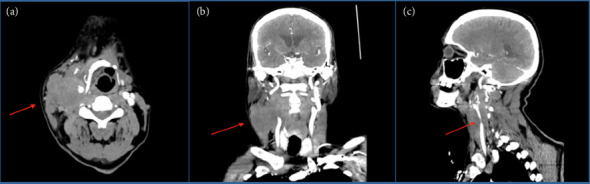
CTA of the neck: (a) transverse view, (b) coronal view, and (c) sagittal view. Red arrows indicate tumor encasement of the right carotid artery.
